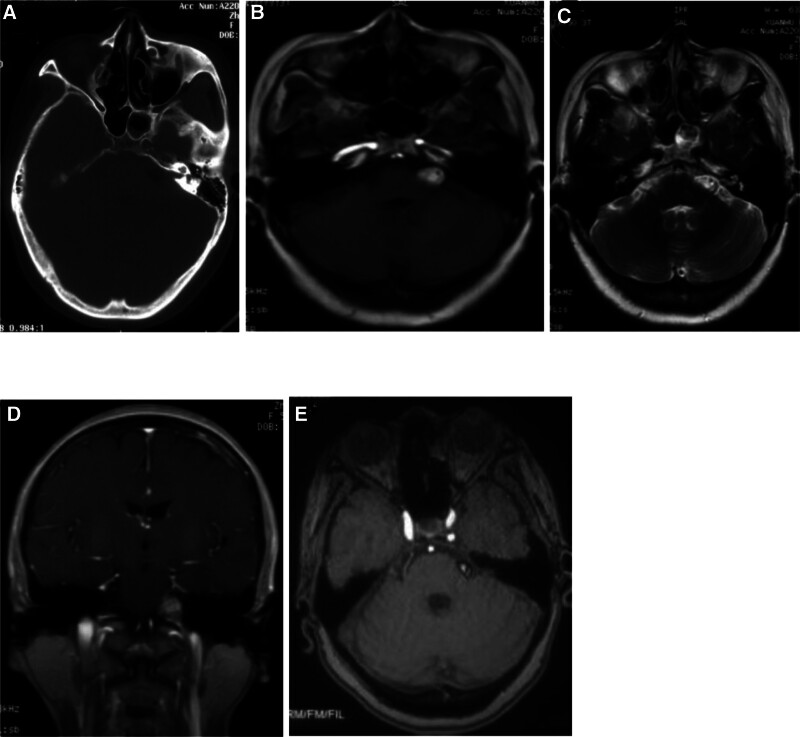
(A) Computed tomography scan of the head shows a hypodense lesion in the left cerebellopontine angle. (B) and (C) Magnetic resonance imaging showing a 1.3 × 0.9 cm lesion in the left cerebellopontine angle area. The mass shows high signal intensity on T1W1 and T2W1 images. (D) The lesion is not enhanced after gadolinium administration. (E) Three-dimensional time-of-flight magnetic resonance angiography showing that the left superior cerebellar artery is adjacent to the trigeminal nerve.
